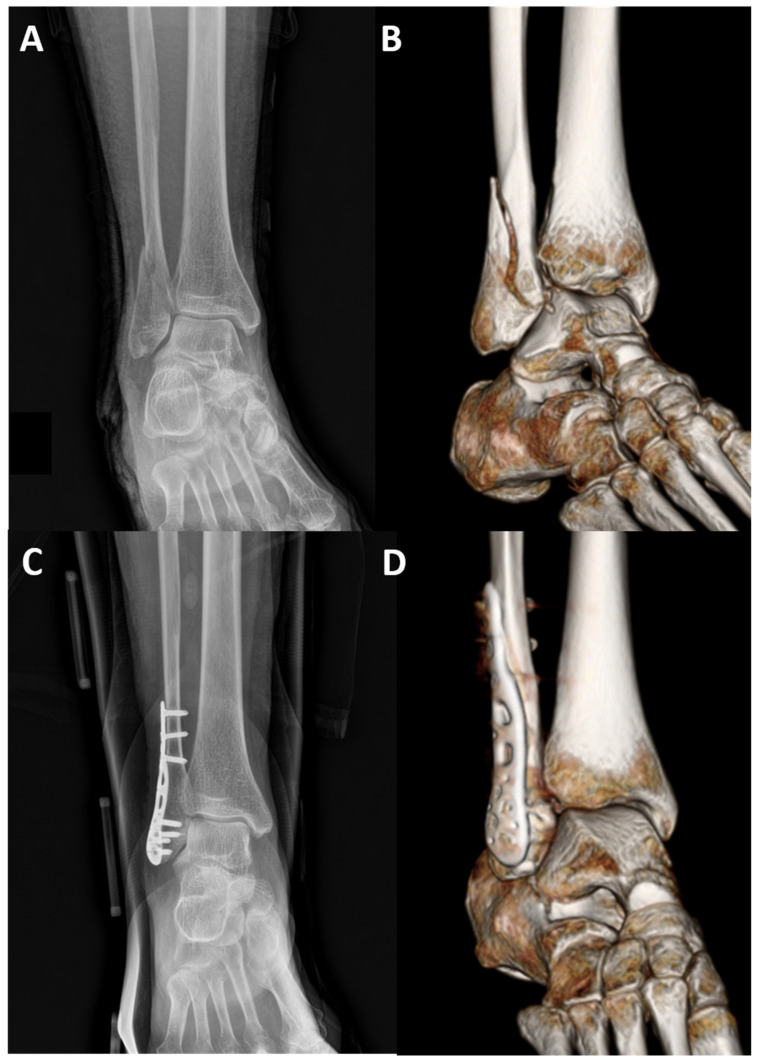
Preoperative radiograph (A) and CT (B) images of an isolated Weber B type fracture. Postoperative radiograph (C) and CT (D) images showing the fracture treated with a three-dimensionally pre-contoured locking plate that is properly fit on the bone.
